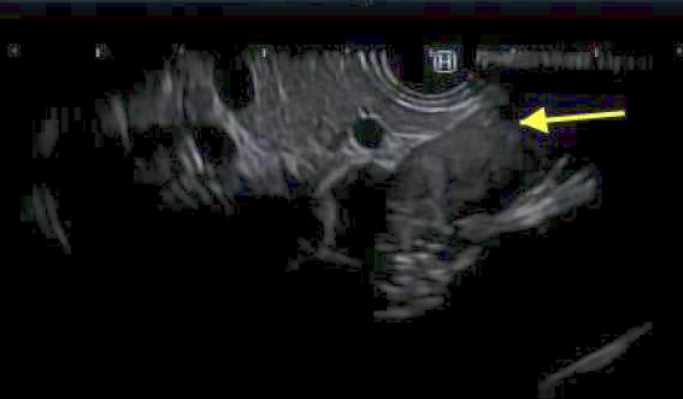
Hypoechoic lesion seen on endoscopic ultrasound images, adjacent to the pancreatic tail and aorta.
